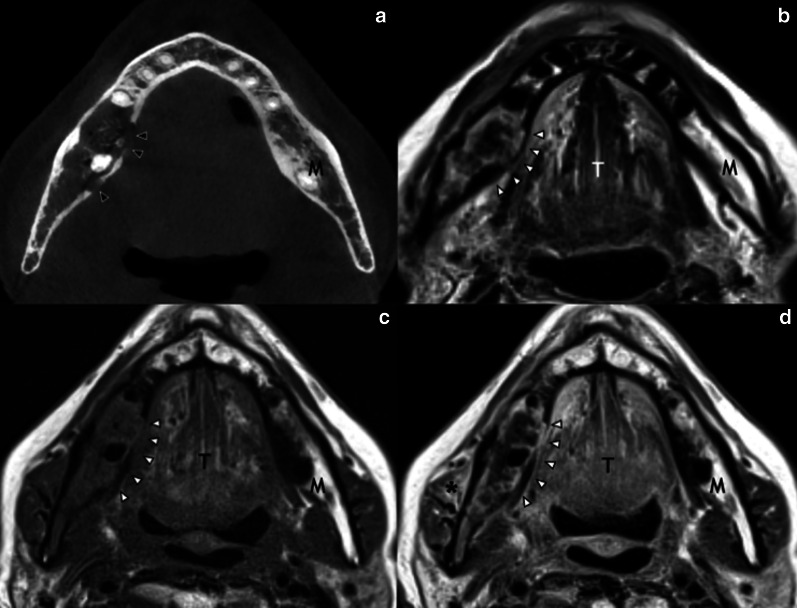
Osteoradionecrosis of the right ramus of the mandible in a 70-year-old woman 4 years after ChT/RT for a right palatine tonsil cancer. Axial CT image (a) shows the loss of spongiosa trabeculation and several cortical breakings (black arrowheads) of the right ramus of the mandible. The corresponding MR slice of the same area (white arrowheads) shows heterogeneous and slightly hyperintense signal on axial T2-weighted (b), with low signal on axial T1-weighted (c), and subtle but diffuse enhancement in the bone and adjacent soft tissues, like the right masseter muscle (asterisk), in post-contrast axial T1-weighted image (d). M, mandible; T, tongue
